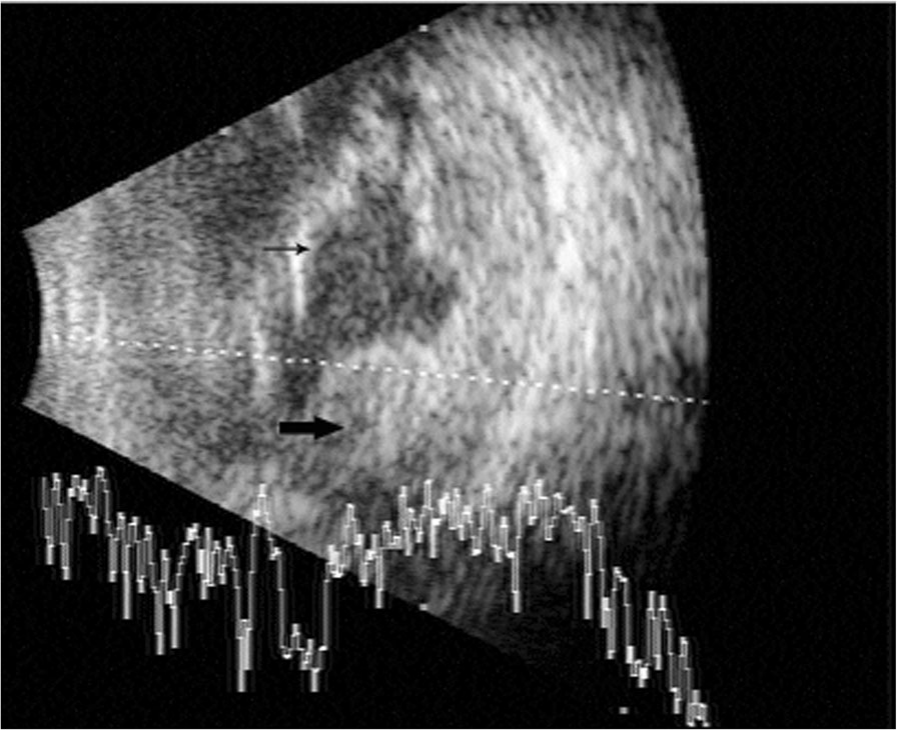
B-scan ultrasound. A vector showing vitreous exudates, the retinal detachment (*thin arrow*), and subretinal abscess (*thick arrow*)
